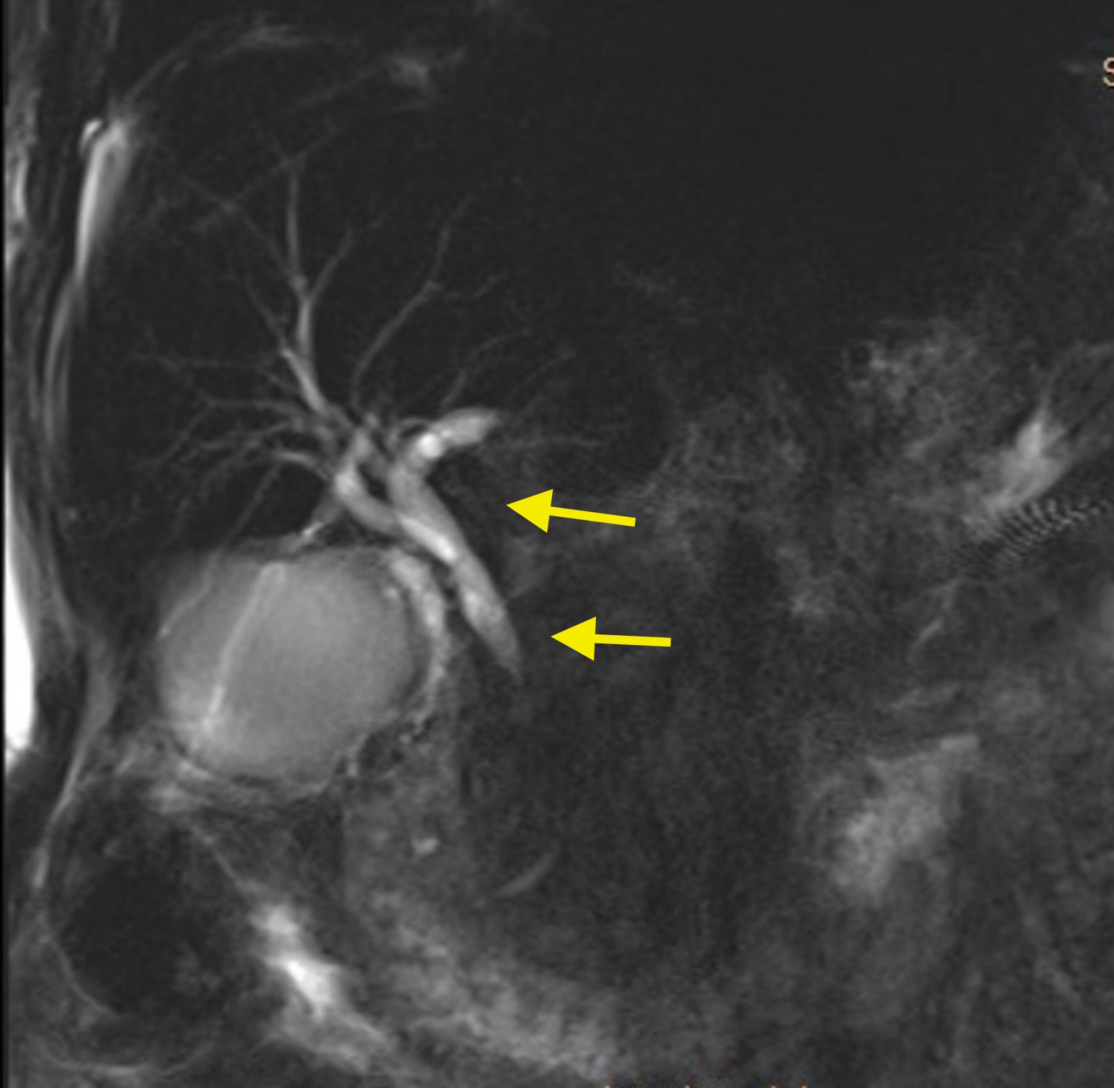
MRI of the abdomen and pelvis showing intra-hepatic bile duct dilation with common bile duct narrowing (arrows) MRI: magnetic resonance imaging
